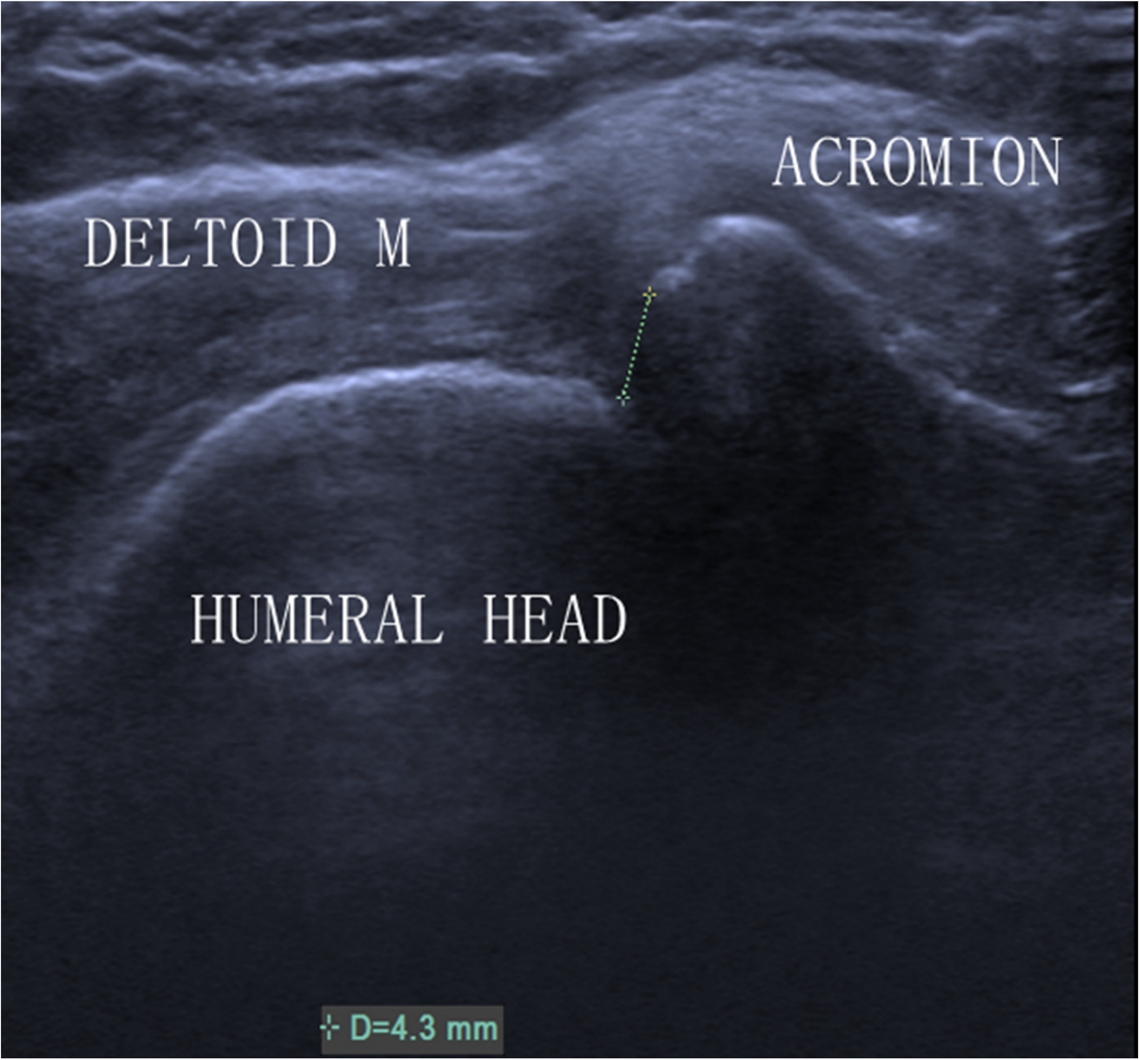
Ultrasound image of AHD in a shoulder with a full-thickness tear of the supraspinatus tendon. M = muscle
